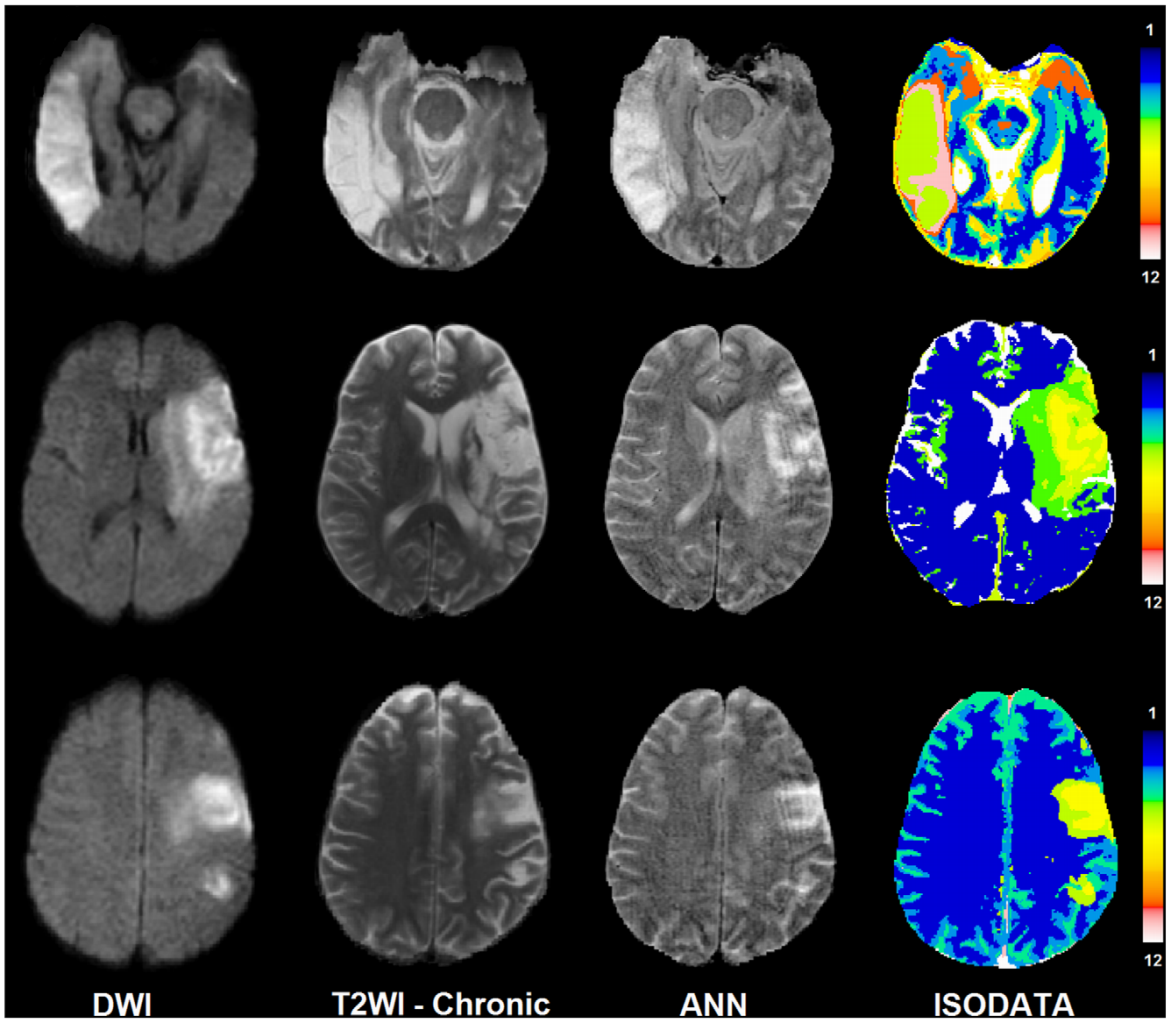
This figure presents DWI, T2WI-Chronic, ANN, and ISODATA maps for 3 different patients. In ISODATA maps the lesions are clustered into 2 to 3 different signatures [from top to bottom: (5, 6, and 8), (2, 3, and 4), (3 and 4)]. Signature number indicates the chance of tissue recovery. The higher the signature values, the lower the chance of recovery.
